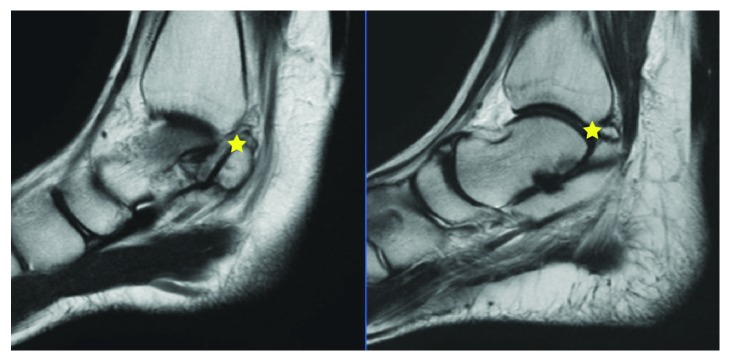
MRI, sagittal view of the ankle; the presence of cartilage between the talar body and the accessory bone can be appreciated (stars).
